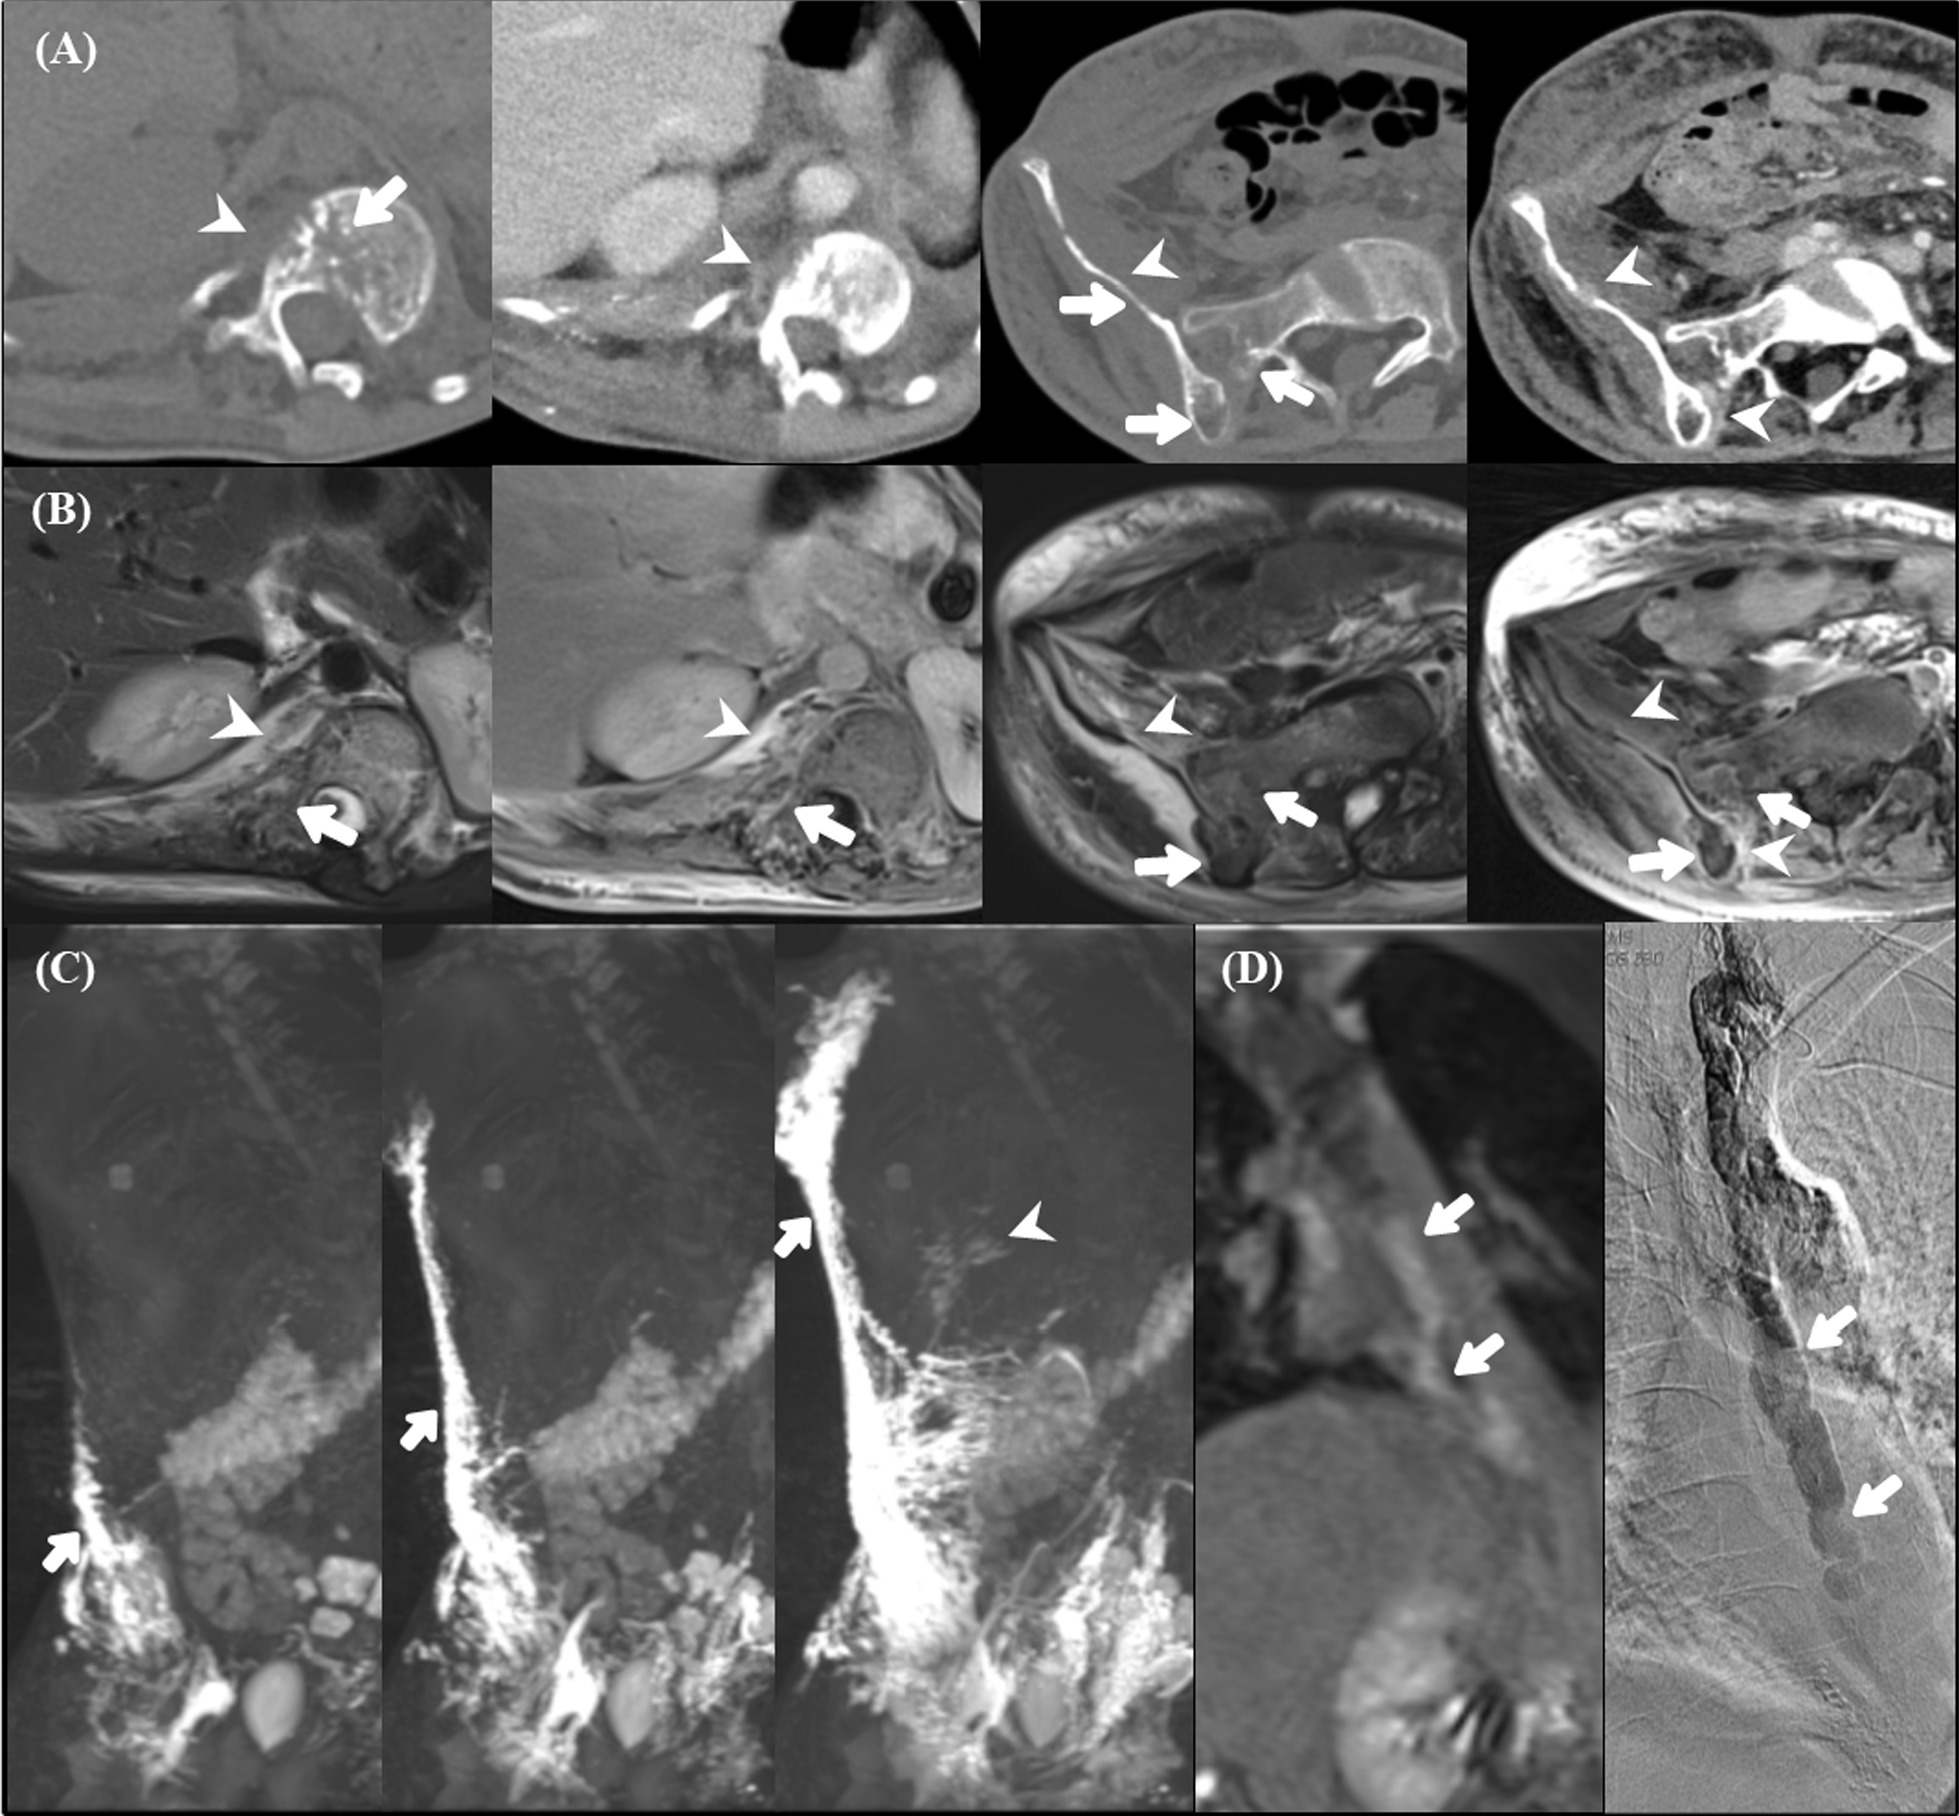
A 14-year-old male with Gorham–Stout disease. A 14-year-old man diagnosed with Gorham‒Stout disease presented with dyspnea related to pleural effusion on the right side. A Non-contrast (first and third columns) and contrast-enhanced (second and fourth columns) computed tomography (CT) images showing osteolytic lesions (arrows) and thickened, enhancing infiltrative soft-tissue lesions (arrowheads) at the lower thoracic
